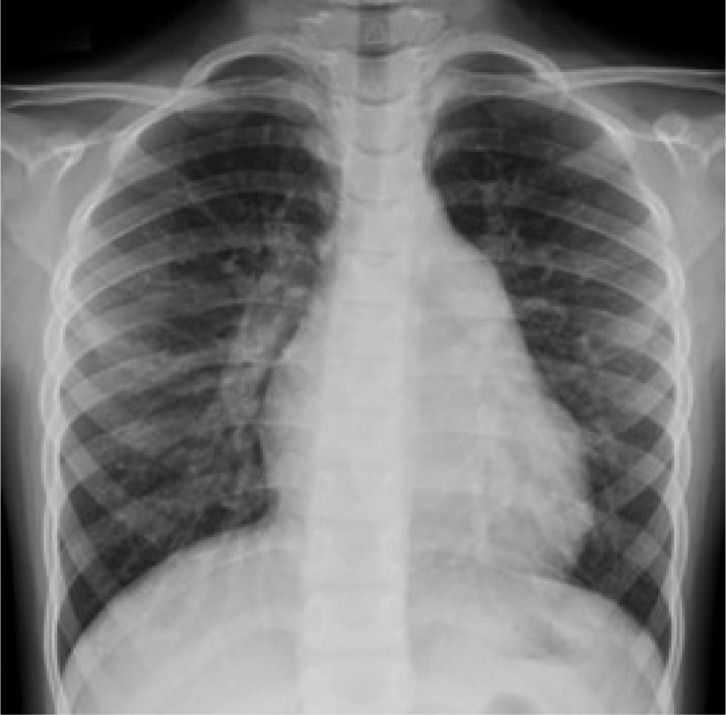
Chest radiography with prominent pulmonary vascular markings and mild
